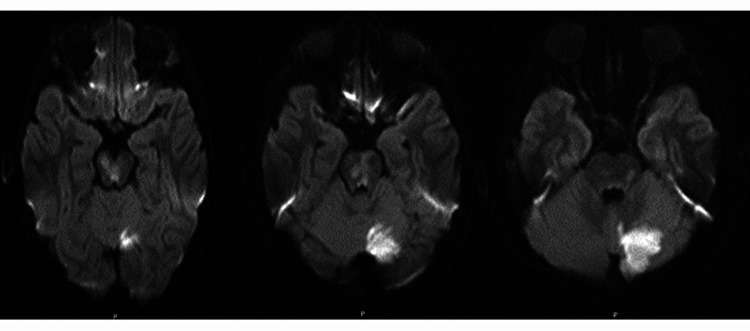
DWI sequence with ischemic changes in the midbrain, pons, and left cerebellum. The hyperintensities noted in the frontal lobes and temporal lobes are susceptibility artifacts. DWI, Diffusion-weighted imaging.
